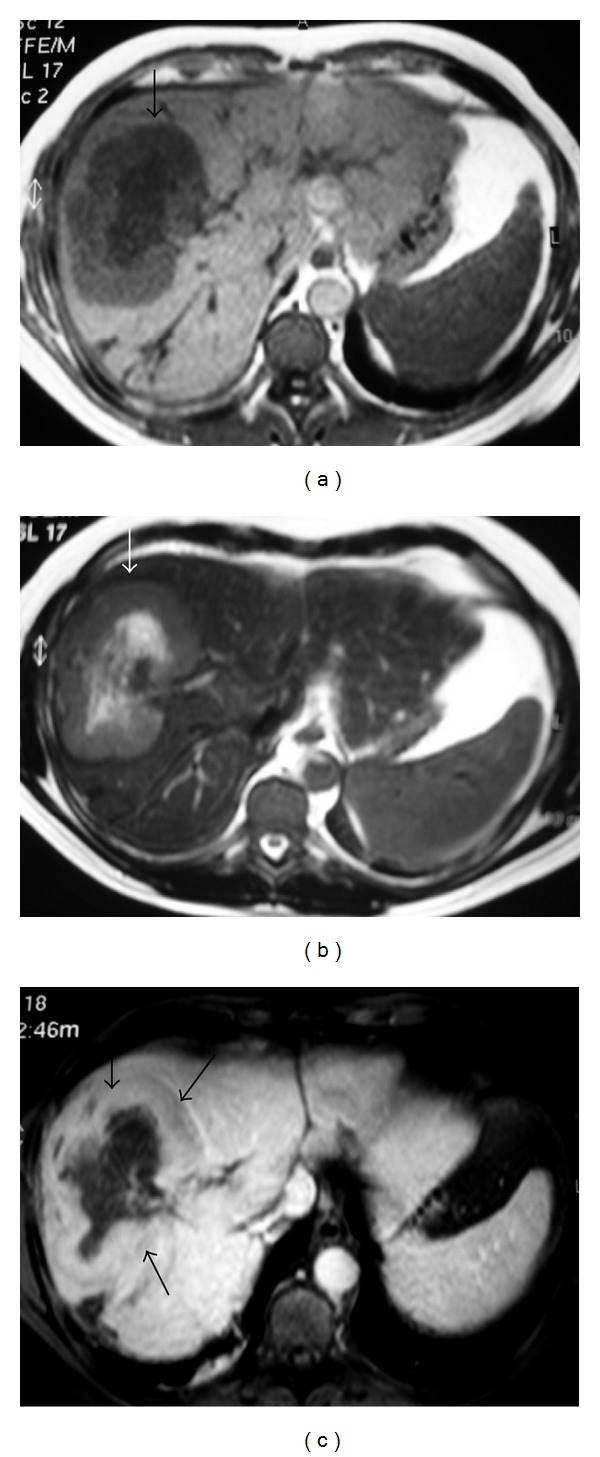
NHL. Axial liver MRI. (a) T1WI and (b) T2WI show heterogeneous mass (arrows) with central area of low signal on T1WI and high signal on T2WI (arrows). (c) The lesion shows enhancement.
